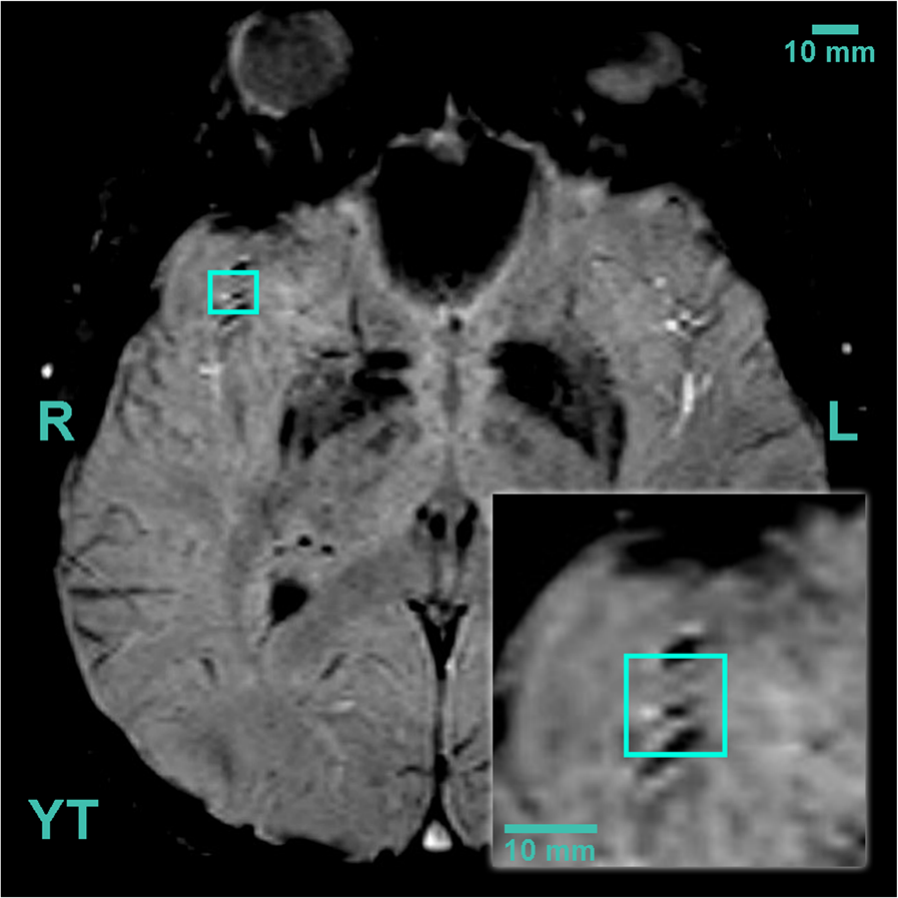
Blue square depicts a cerebral microbleed (CMB) in the right inferior longitudinal fasciculus of a young TBI patient (YT) (40-year-old male, mild TBI). On the axial susceptibility-weighted magnetic resonance image (SWI, obtained at 3 Tesla), the bleeding appears as an ovoid, hypointense lesion [20, 32, 33]
